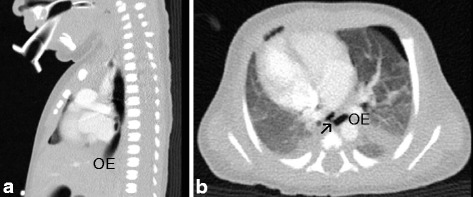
a Sagittal chest CT scan showing long-segment agenesis of the trachea, only oesophagus (OE) visible. b Horizontal chest CT scan displaying the fistula (arrow ↗) from the oesophagus (OE) to a ventral distal tracheal pouch (blind proximal ending) at the level of thoracic vertebrae 4–5
